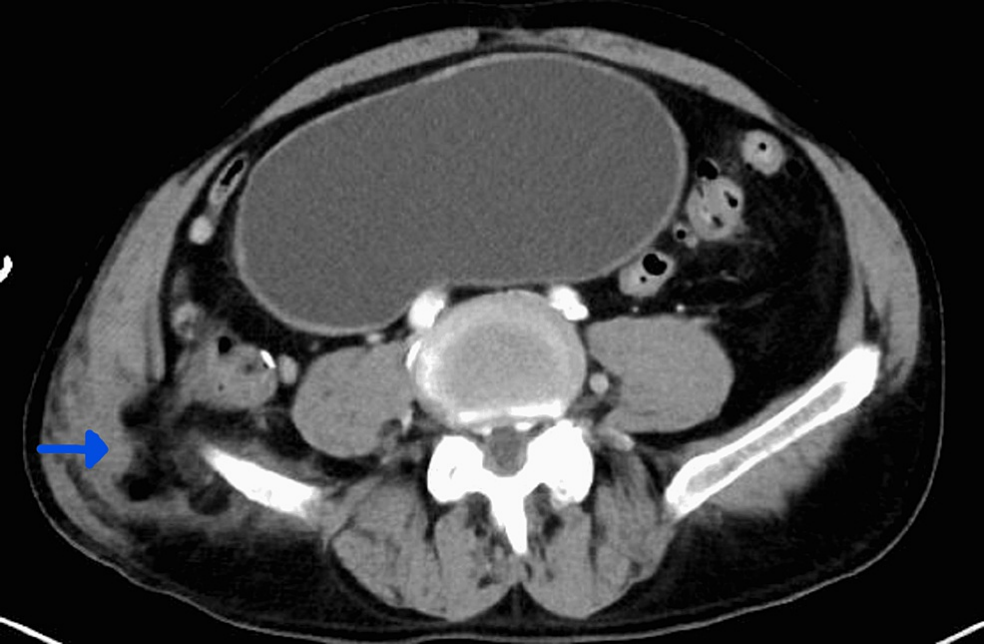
Initial CT of the abdomen/pelvis demonstrating a right lumbar defect containing fat (see blue arrow)
